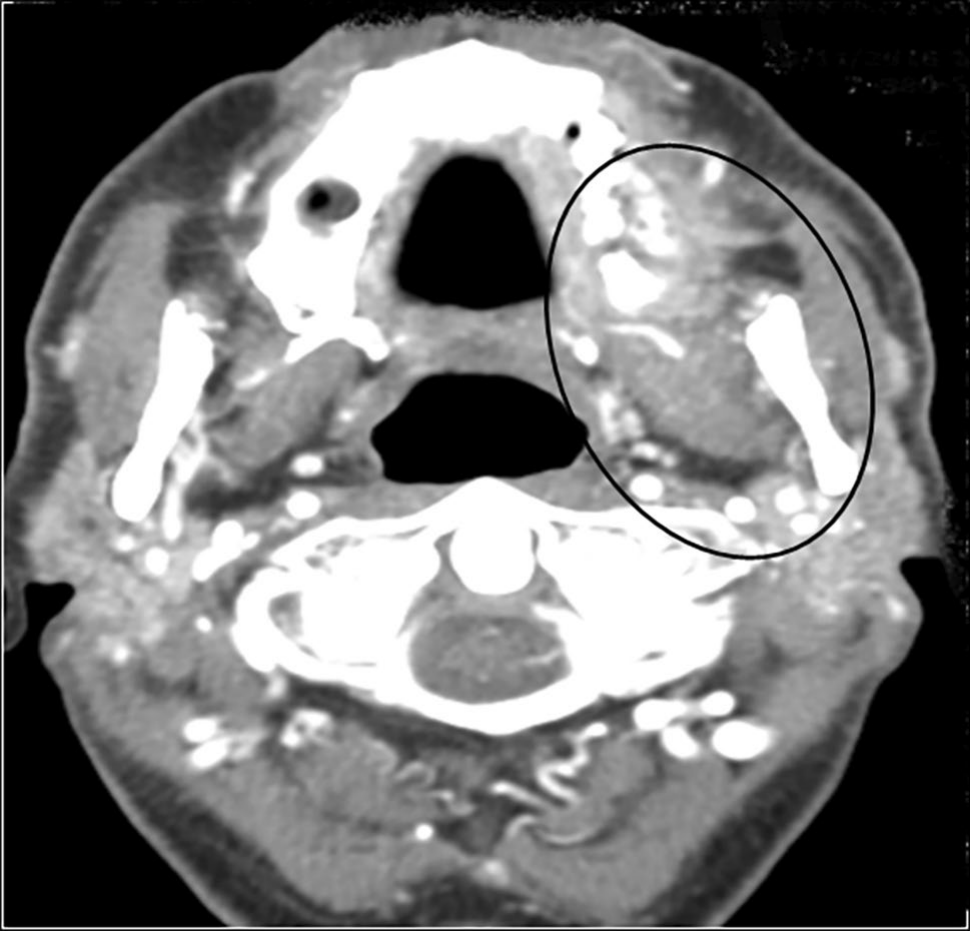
CT scan of the skull image showing an inflammatory morphological pattern of the left maxillary bone with bone erosion, in communication with the oral cavity. The inflammatory lesion involved the contiguous soft tissues too
